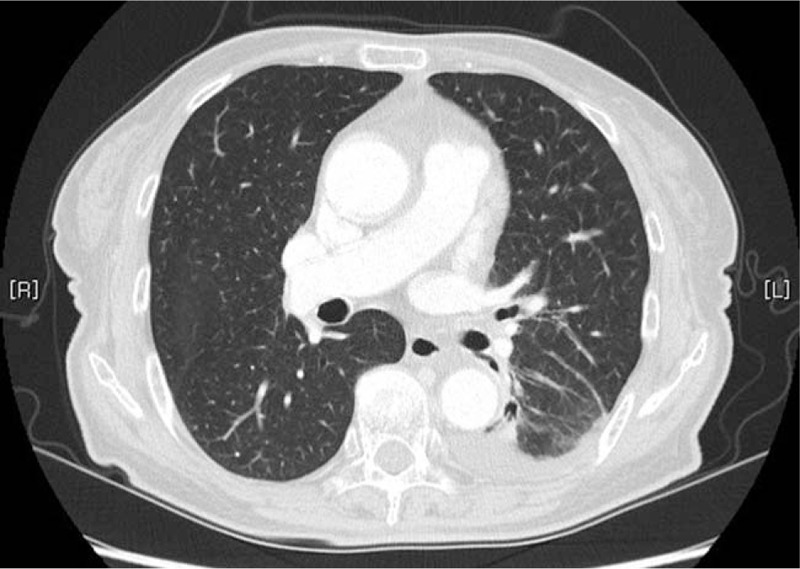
A follow-up chest computed tomography scan on April 17, 2014, indicates a decrease in the mass, with the longest axis, of 1.5 cm, in the left lower lobe and a decrease in the extent of lymphangitic metastasis and pleural effusion.
